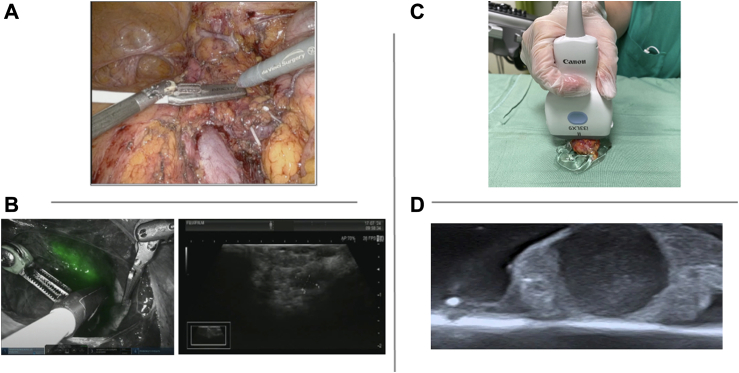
A-B) intraoperative robotic ultrasound with drop-in probes; A) drop-in probe, in vivo analysis of a sentinel lymph node B) near-infrared visualization with indocyanine green highlights and ultrasound images. C–D) High-frequency ultrasound C) ex vivo analysis of a pelvic lymph node; D) ultrasound appearance of a metastatic lymph node.
